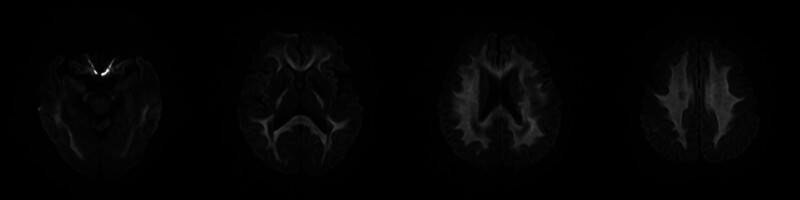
Brain diffusion-weighted MRI during the acute stages of fluoroacetamide intoxication in a 58-year-old man. Abnormal signals in the cerebral peduncles, corpus callosum, internal and external capsules, and cerebral white matter. MRI = magnetic resonance imaging.
